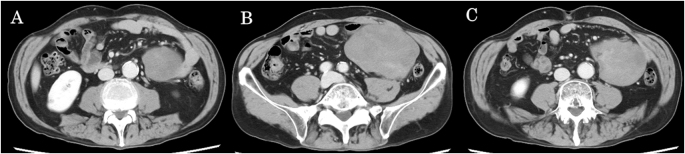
(A) Contrast-enhanced computed tomography images show an intra-abdominal mass adjacent to the ileum. (B, C) The mass was a large, well-circumscribed solid lesion measuring approximately 12 × 10 cm, with mild-to-moderate heterogeneous enhancement.
